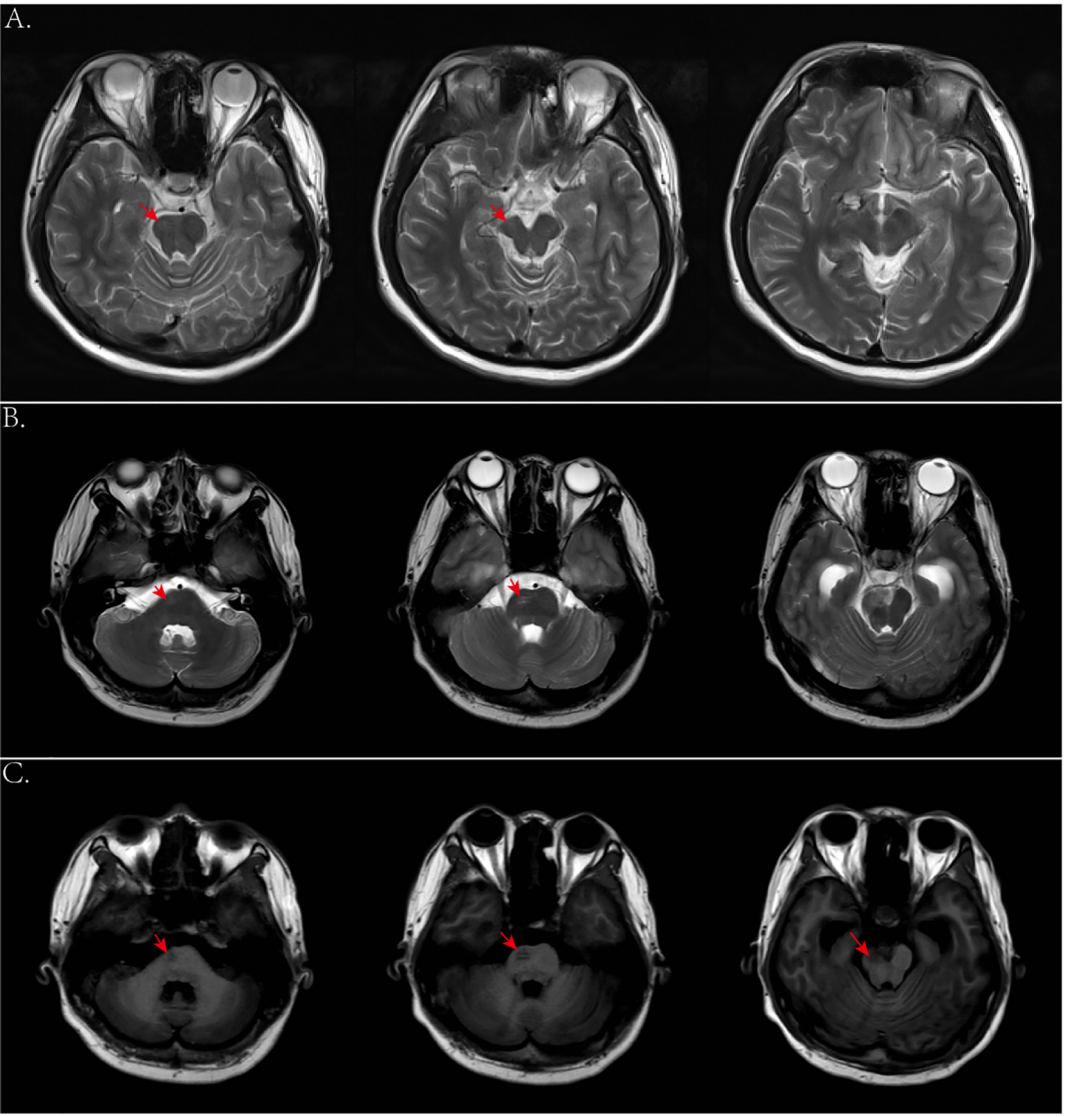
(A) Axial T2-weighted images 2 years ago showed mild atrophy of the brainstem on the affected side. (B, C) Axial T2- and T1-weighted images this time showed degeneration and damage of the brainstem conduction fiber bundles on the affected side.
